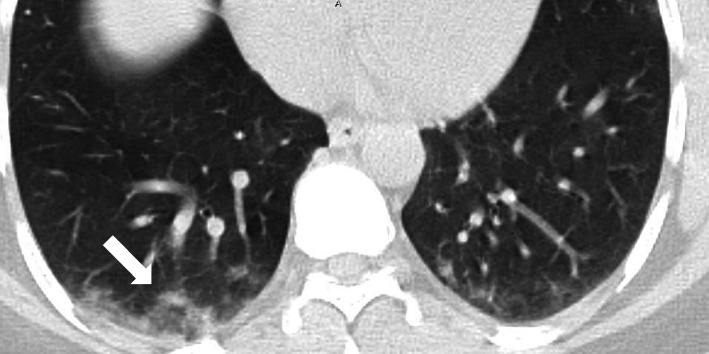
A 37-year-old female patient with COVID-19 with 4 days of fever cough and dyspnea, CT chest axial image shows predominantly subpleural ground glass opacities with vascular thickening and shows a right lower lobe subpleural opacity with “reversed halo” sign (arrow)
